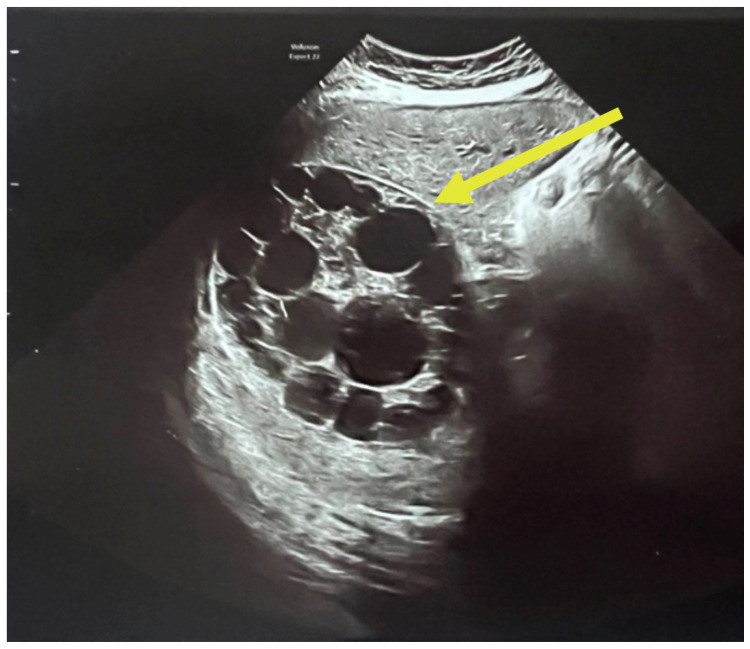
Ultrasound of hydatid cyst with multiple daughter cysts: large, well-defined hydatid cyst with internal multiple daughter vesicles—a hallmark of *Echinococcus granulosus* infection. The cyst shows a “rosette” or “honeycomb” appearance, characteristic of CE2-stage cysts per WHO classification [23].
